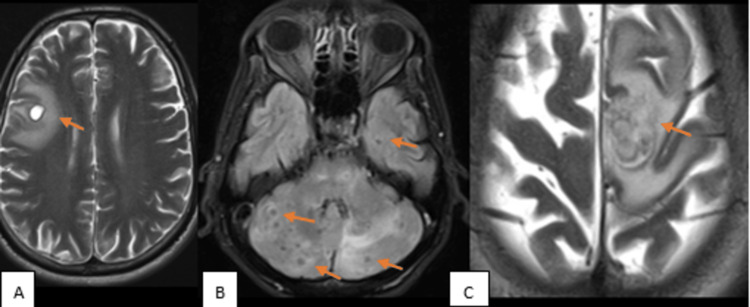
MRI images demonstrating the number of lesions in our study cases (A) MRI axial T2-weighted imaging (T2WI): A single lesion (orange arrow) was noted in the right frontal lobe - a case of neurocysticercosis. (B) MRI axial fluid-attenuated inversion recovery (FLAIR): Multiple lesions (orange arrows) in the bilateral cerebellar hemisphere and left temporal lobe - a case of tuberculoma. (C) MRI axial T2WI: Conglomerate lesion (orange arrow) in left high parietal lobe - a case of tuberculoma.
